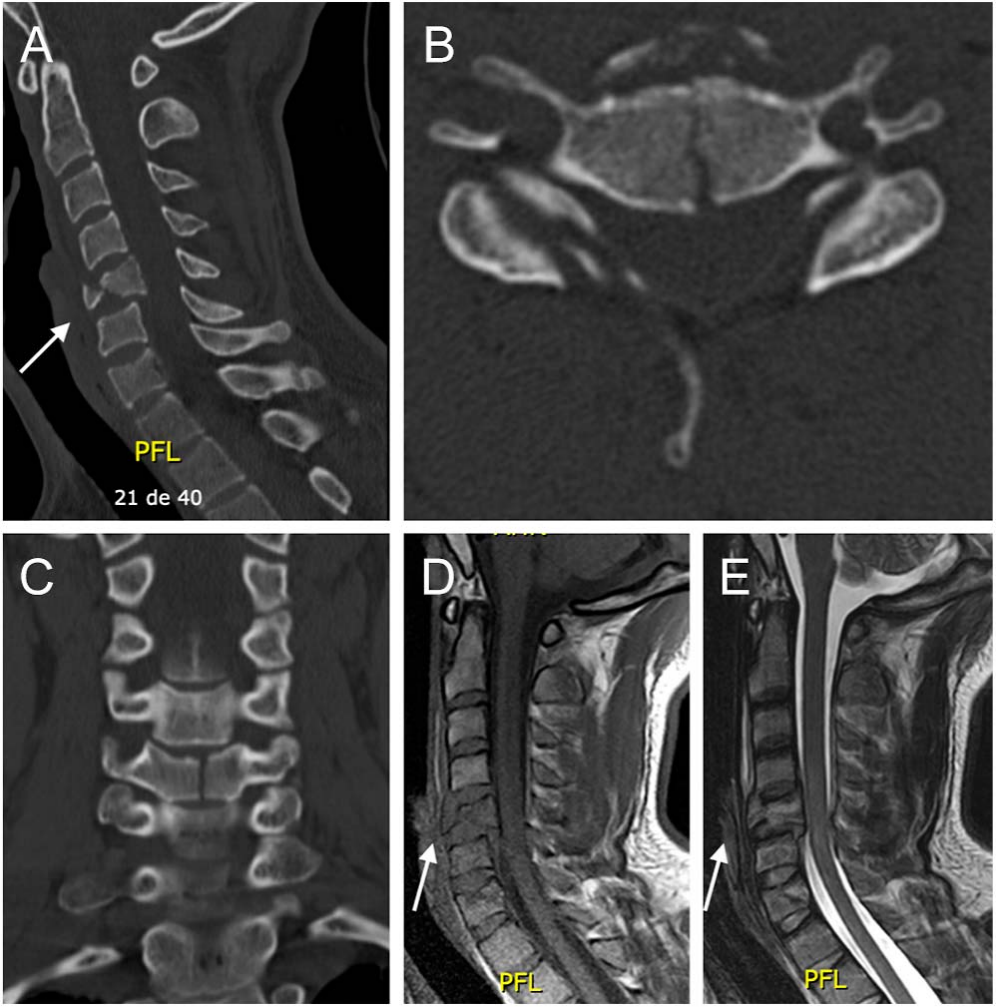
Sagittal (A), axial (B), and coronal (C) CT scans showing a C5 teardrop fracture. Sagittal reconstruction using MRI in T1 (D) and T2 (E) sequences of the same lesion, showing spinal cord signal changes and posterior disc and PLL disruption.
